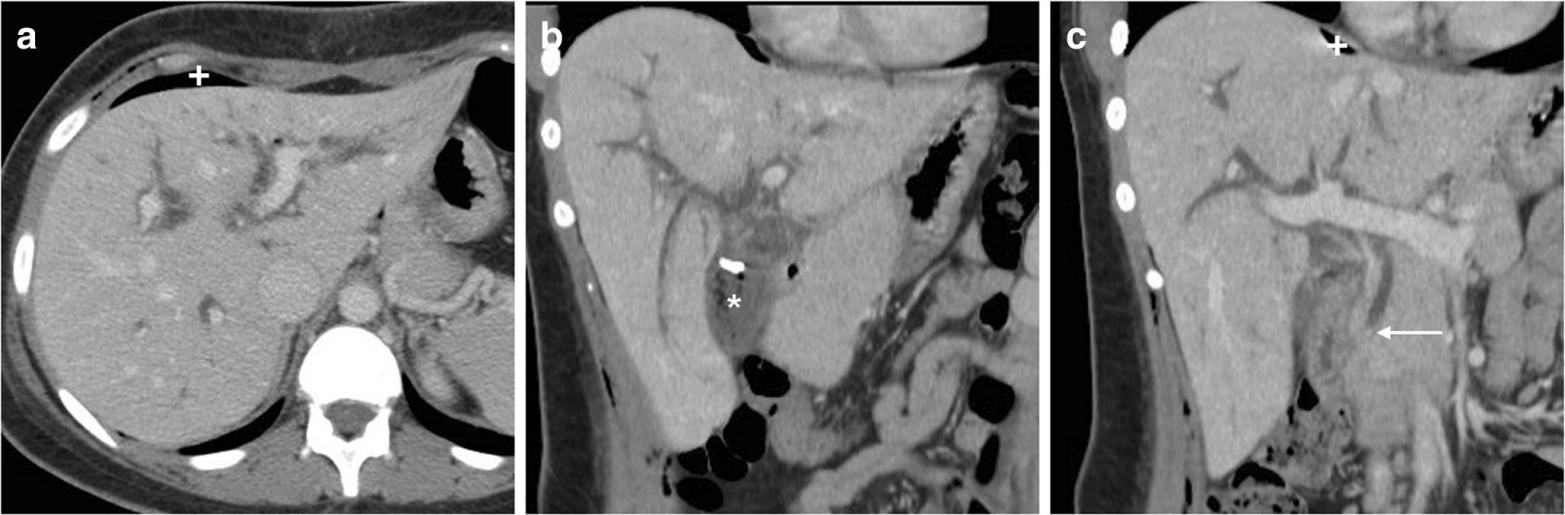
Residual lithiasis and cholangitis after laparoscopic cholecystectomy, developing despite preoperative ERCP. On the 6th postoperative day, CT (a–c) showed residual intraperitoneal air (+ in a), usual collection at gallbladder fossa (* in b), “mottled” liver parenchymal enhancement and bilateral dilatation of intrahepatic bile ducts. Focused coronal image (c) showed a tiny stone (*thin arrow*) at the distal CBD, confirmed and treated by ERCP
